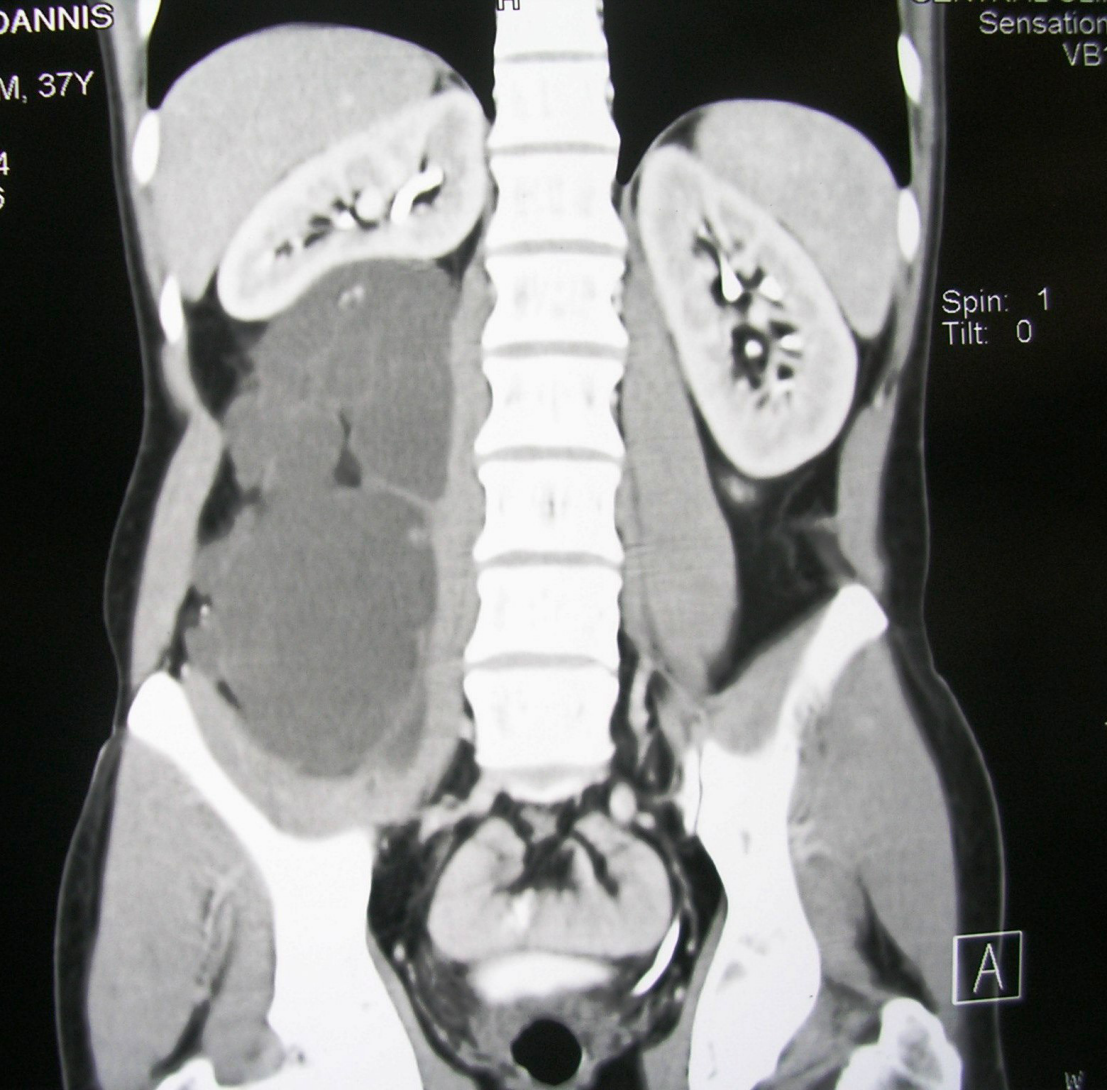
Coronal CT image describing the size of the tumor and its mass effect to the right kidney.
